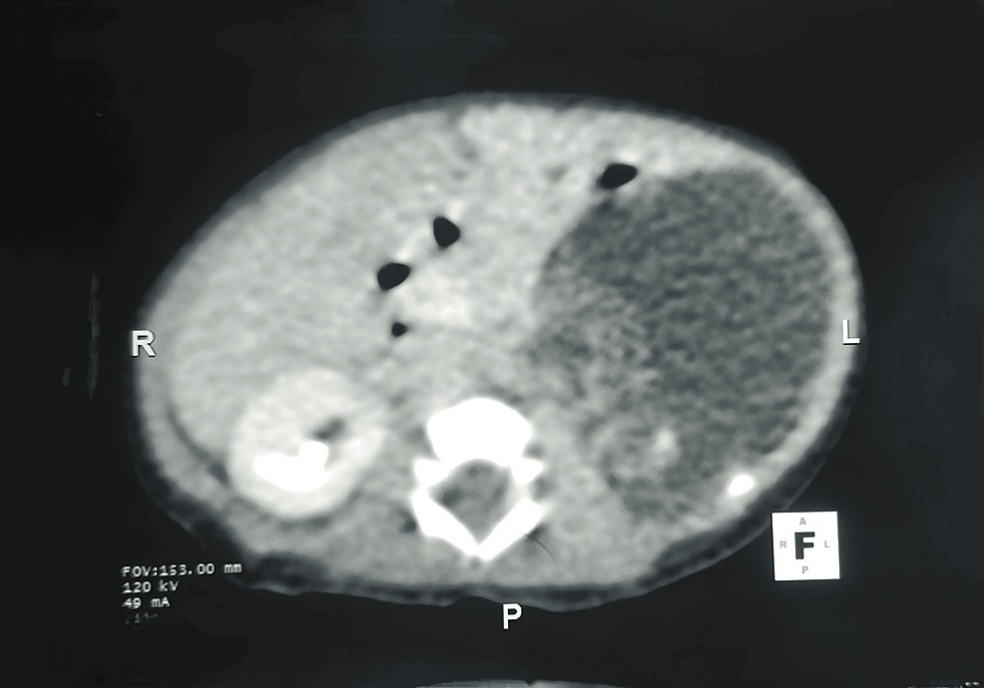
Contrast-enhanced computed tomography of abdomen depicting a huge retroperitoneal cystic mass lesion. A large cystic mass lesion was noted in the retroperitoneum on left side in suprarenal location with few calcifications and a solid component within the cystic lesion.
